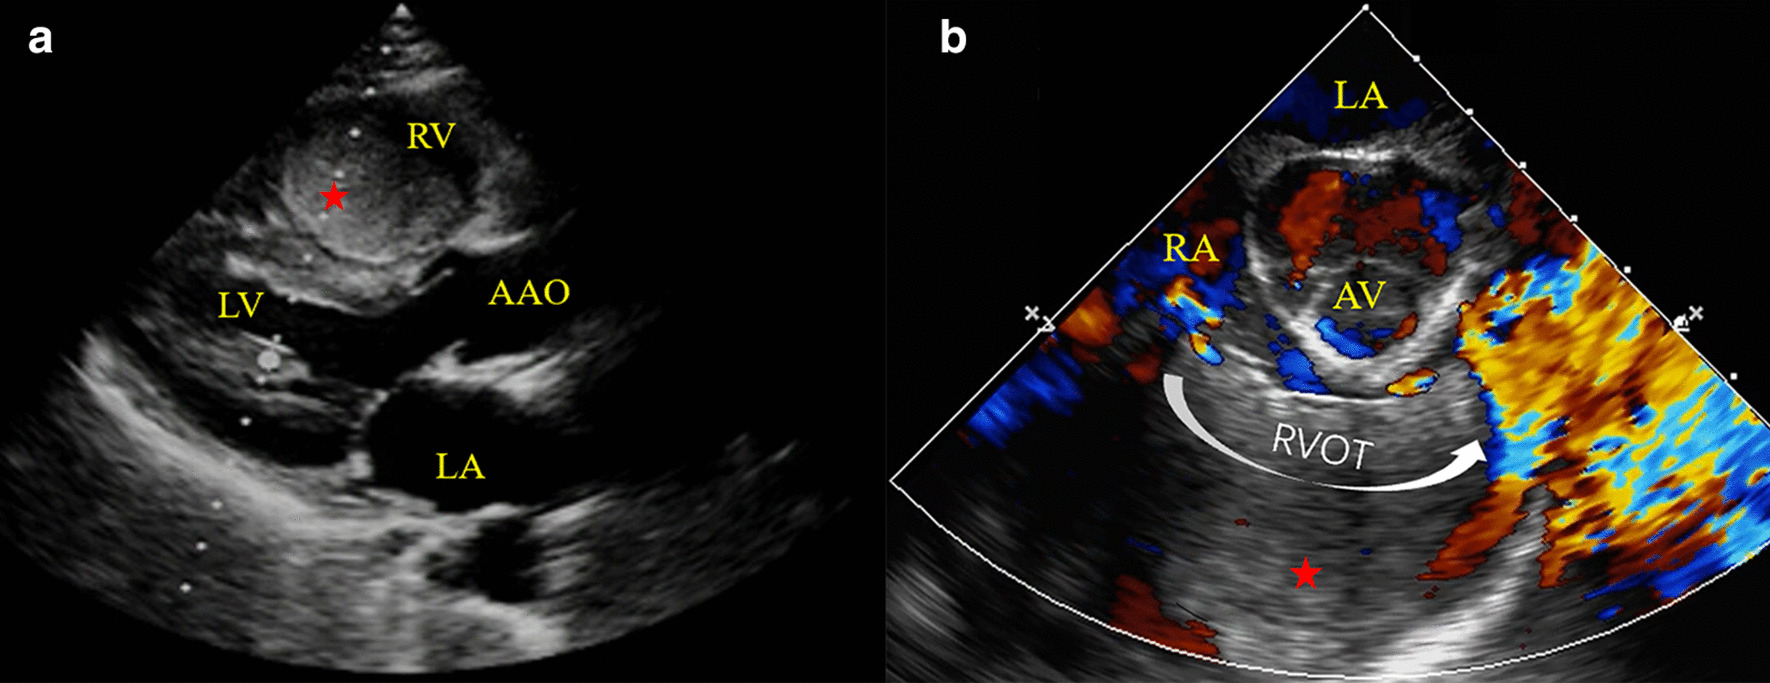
a transthoracic echocardiography before surgery showed a giant tumor (red star) occupied the majority of RV; b Transesophageal echocardiography (ME RV inflow-outflow tract) showed the tumor (red star) in right ventricular caused outflow obstruction. *LA* left atria, *LV* left ventricle, *RA* right atria, *RV* right ventricle, *AAO* ascending aorta, *AV* aortic valve, *RVOT* right ventricular outflow tract
